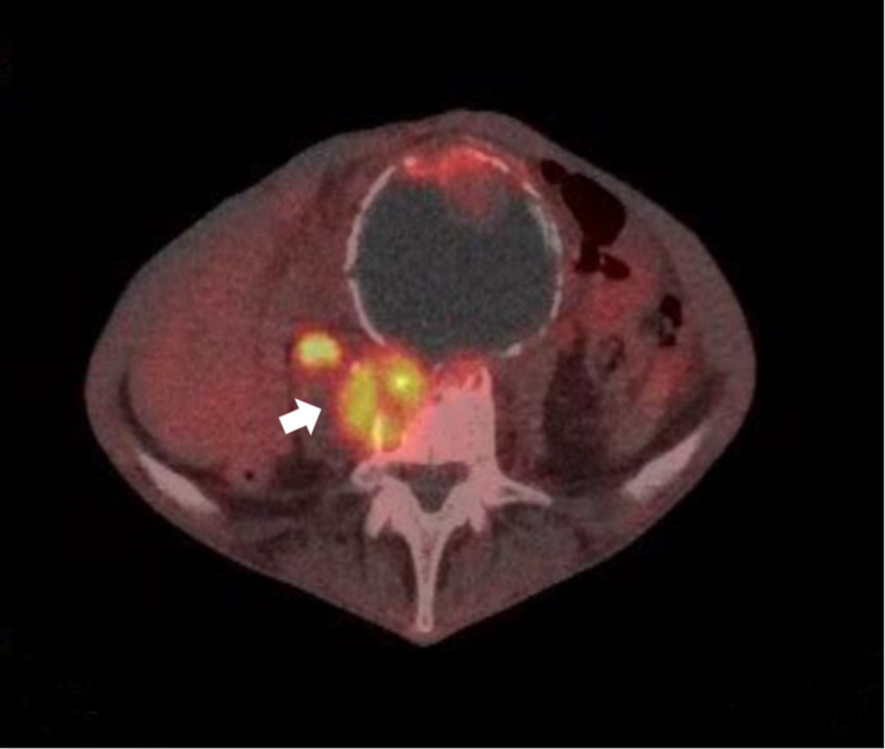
FDG-PET scan showing L4/5 disc destruction and intense FDG uptake within the disc with increased activity in the area of extension to the right psoas muscle (arrow). FDG, fludeoxyglucose.
